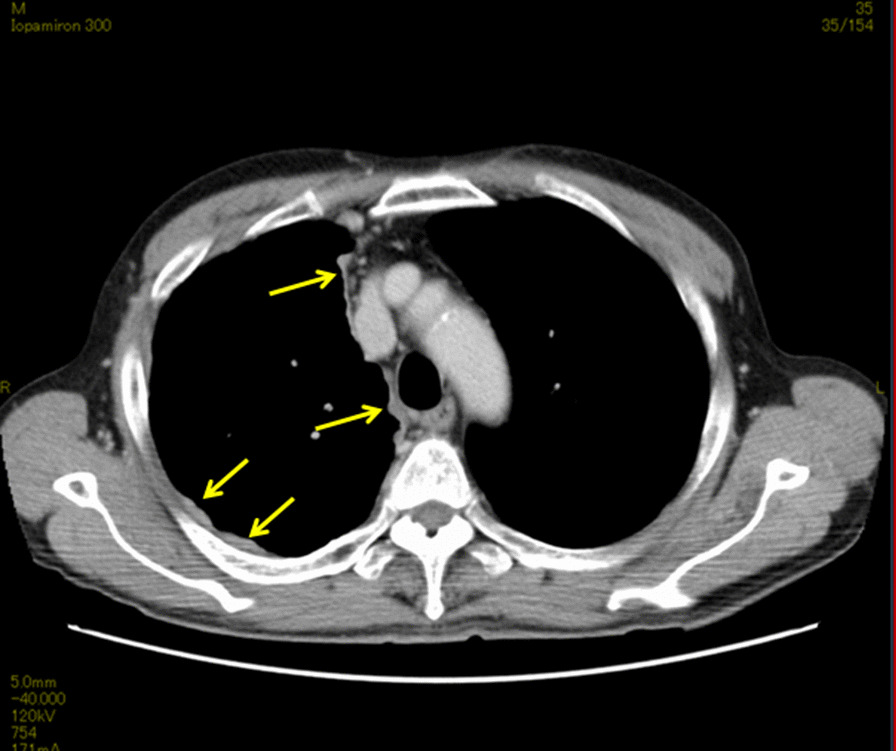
Images showing chest computed tomography (CT) scans. The yellow arrows indicate the diffuse thickened pleura in the right chest cavity. The shape of thickened pleura is also irregular
